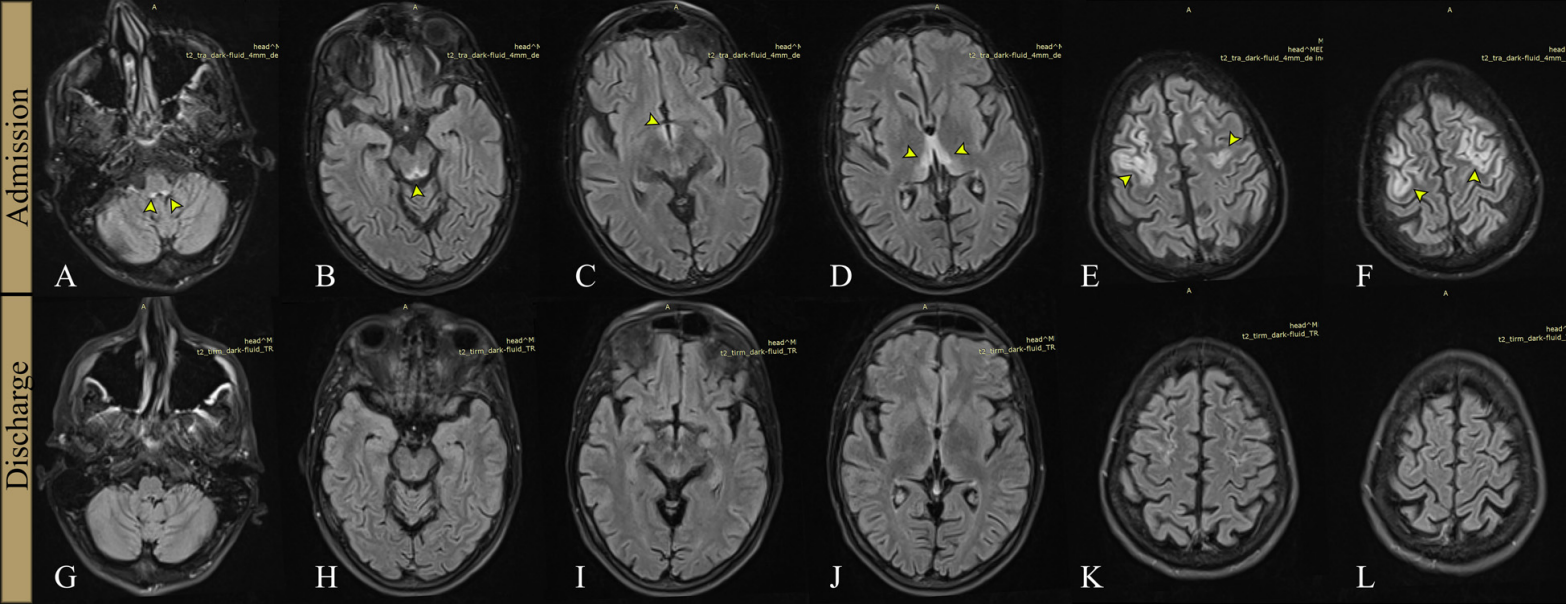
Cerebral MRI examinations at admission and discharge. FLAIR sections at admission (A–F), respectively at discharge, 8 weeks later (G–L). Hyperintense signal (arrows) is visible on the first examination, in the medullo-pontine tegmentum (A), around the aqueduct of Sylvius (B), in the mamillary bodies (C), medial thalami (D), and in the frontal cortex bilaterally (E, F). The second examination (G–L) shows marked improvement of the signal changes in initially affected areas.
